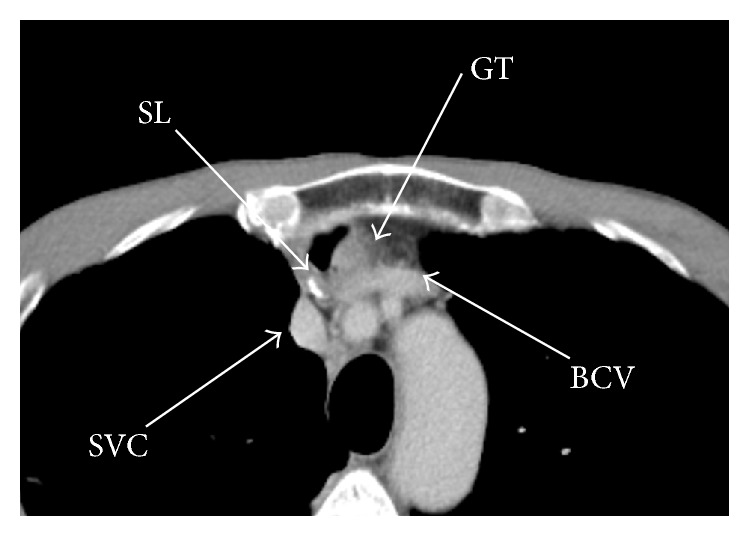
Computed tomography of the anterior mediastinum. GT: gastric tube, SL: staple line, SV: superior vena cava, and BCV: brachiocephalic vein.
